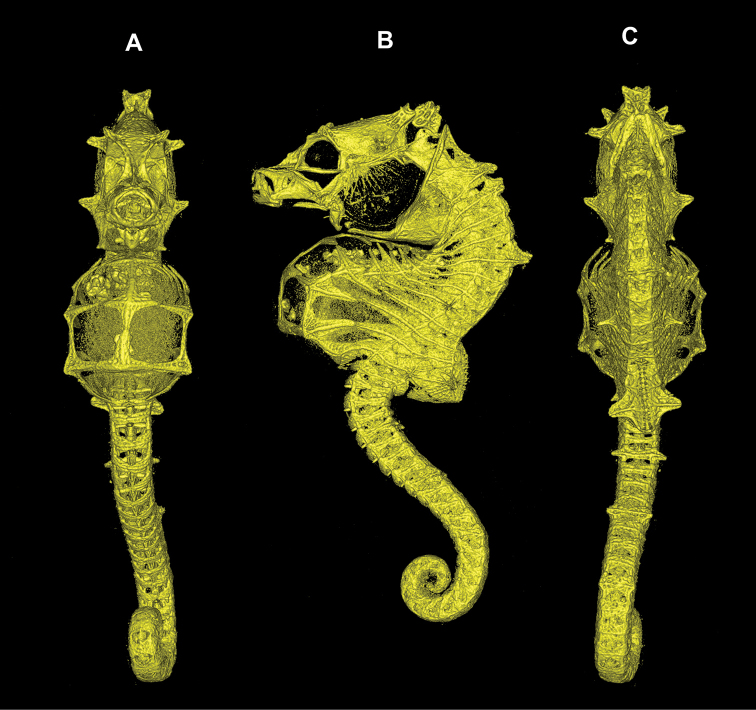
Computed tomography scanned skeleton of *Hippocampus
